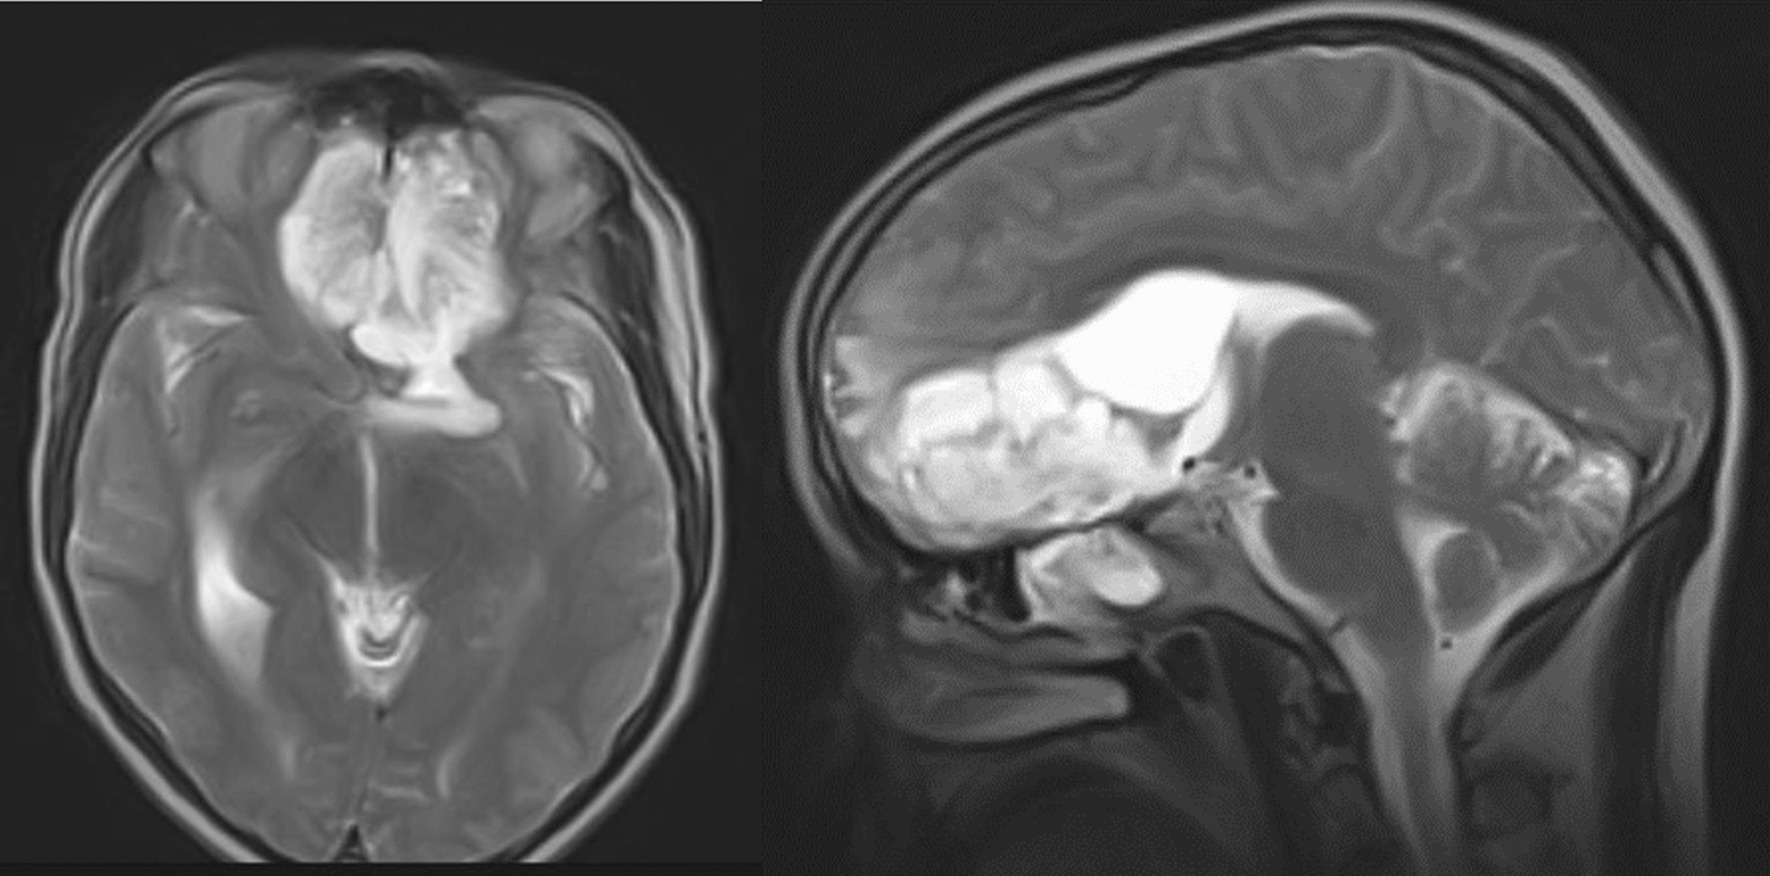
Preoperative T2-weighted images axial and sagittal images demonstrating hyper intense lesion with perilesional edema at olfactory groove region
